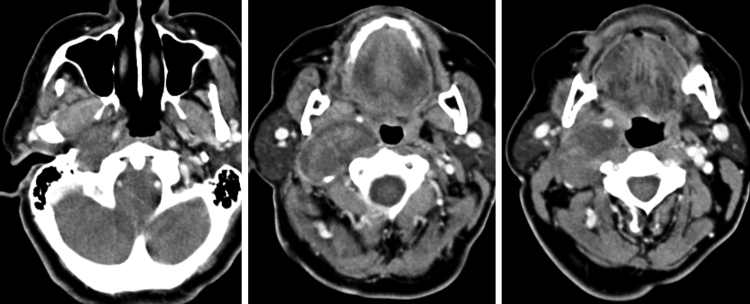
Valgus nerve schwannoma: axial computed tomography shows a heterogeneous mass with well-defined limits, displacing the internal carotid artery and internal jugular vein.
